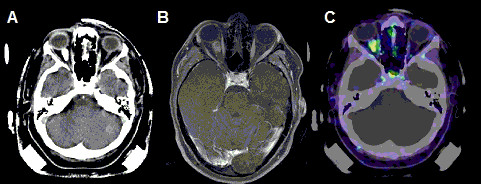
Treatment-planning examinations for a patient with right-sided ONSM for primary FSRT. CT (A), MRI (B) and 86-Ga-DOTATOC-Pet (C) were used for treatment planning after image-fusion, a dose of 54 Gy was prescribed in 1.8 Gy single doses.
